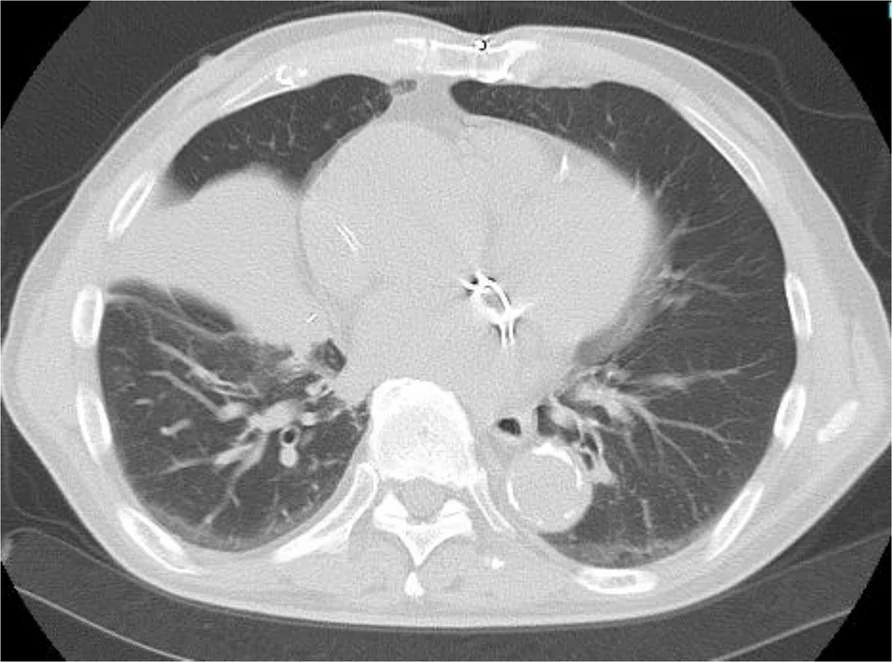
Chest computed tomography showing right middle lobe atelectasis
